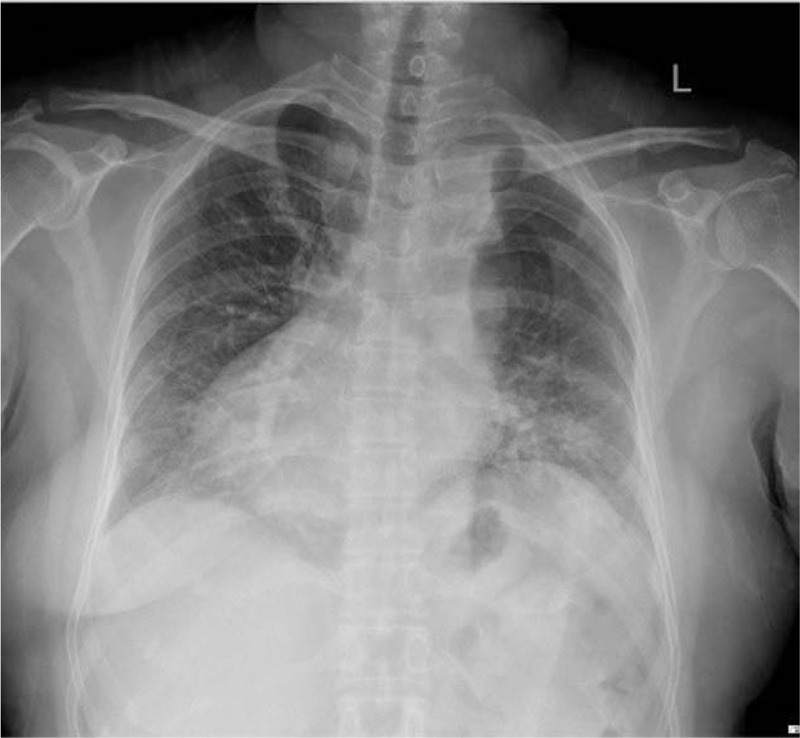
Chest X-ray: dextrocardia.
